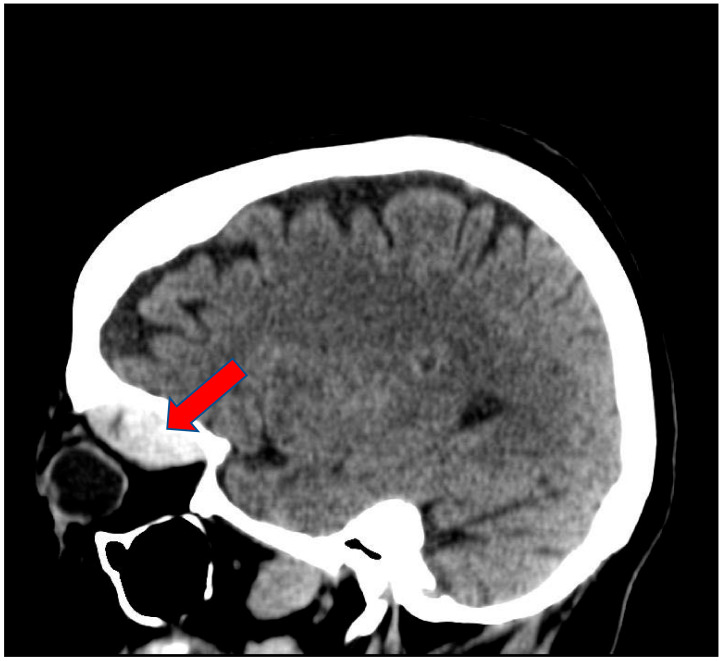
Sagittal view of Brain CT scan showing left soft tissue swelling with hyper dense mass in the left superior orbit measuring 3.7 × 2.9 × 1.5 cm (Arrow).
